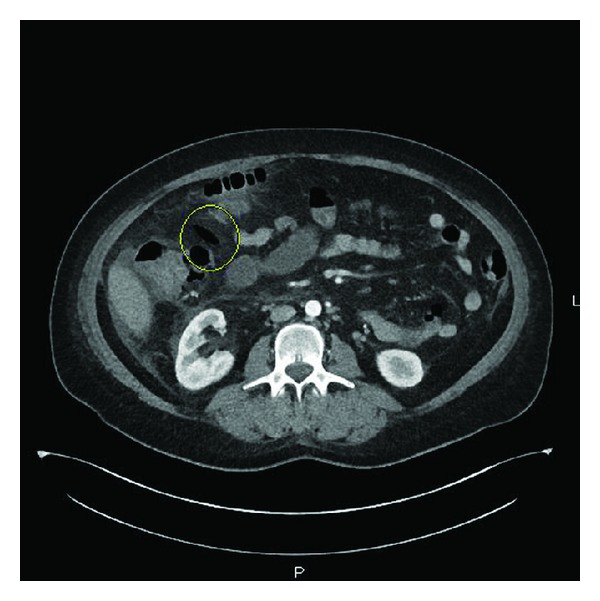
Contrast-enhanced CT abdomen showing wall thickening in the ascending colon with pericolonic stranding pattern and adjacent free air (circle) suggestive of colon perforation.
